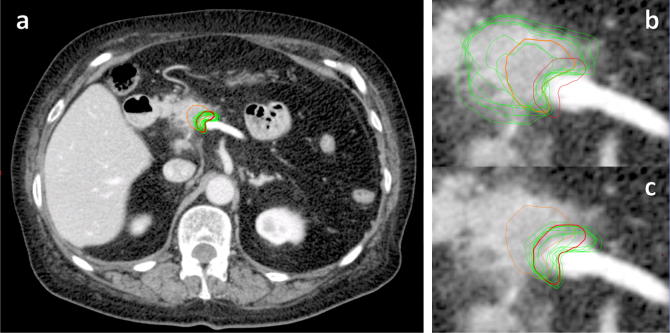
Reference (orange = GTV, red = boost volume) and investigator contours (green) of boost volume (images a and c) and GTV (image b) on contrast-enhanced axial CT of patient with borderline-resectable pancreatic carcinoma.
